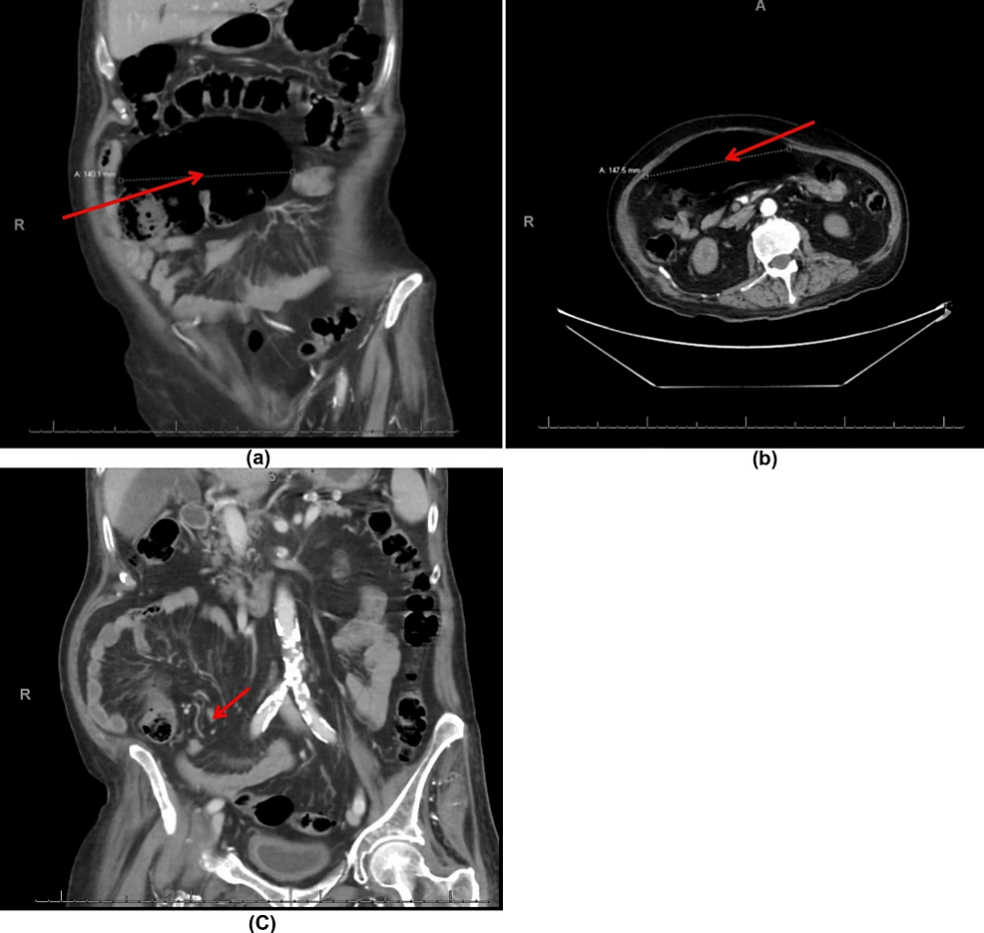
CT abdomen with IV contrast showing a twist in the bowel, mesentery, and associated vessels ''whirlpool sign'', and a grossly dilated cecum of 14 cm diameter (a) Coronal view showing the hugely dilated cecum; (b) Transverse view; (c) Coronal view showing the ''whirlpool sign'' with torsion of the cecal vessels
